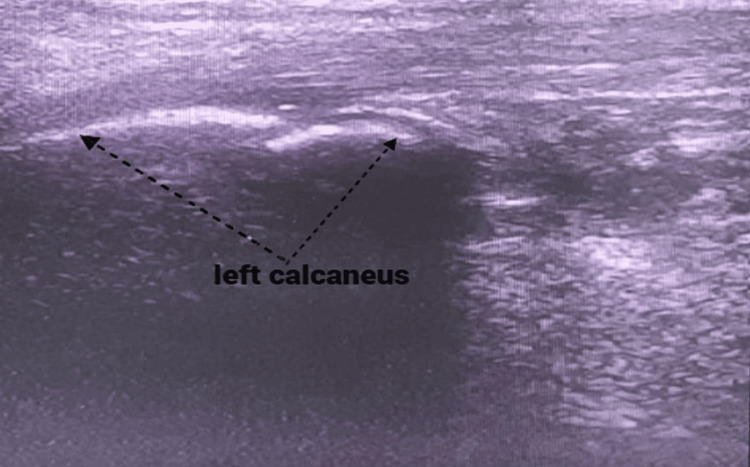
Ultrasound of the left heel longitudinal section showing the presence of fragmentation of the calcaneal apophysis consistent with Sever's disease
